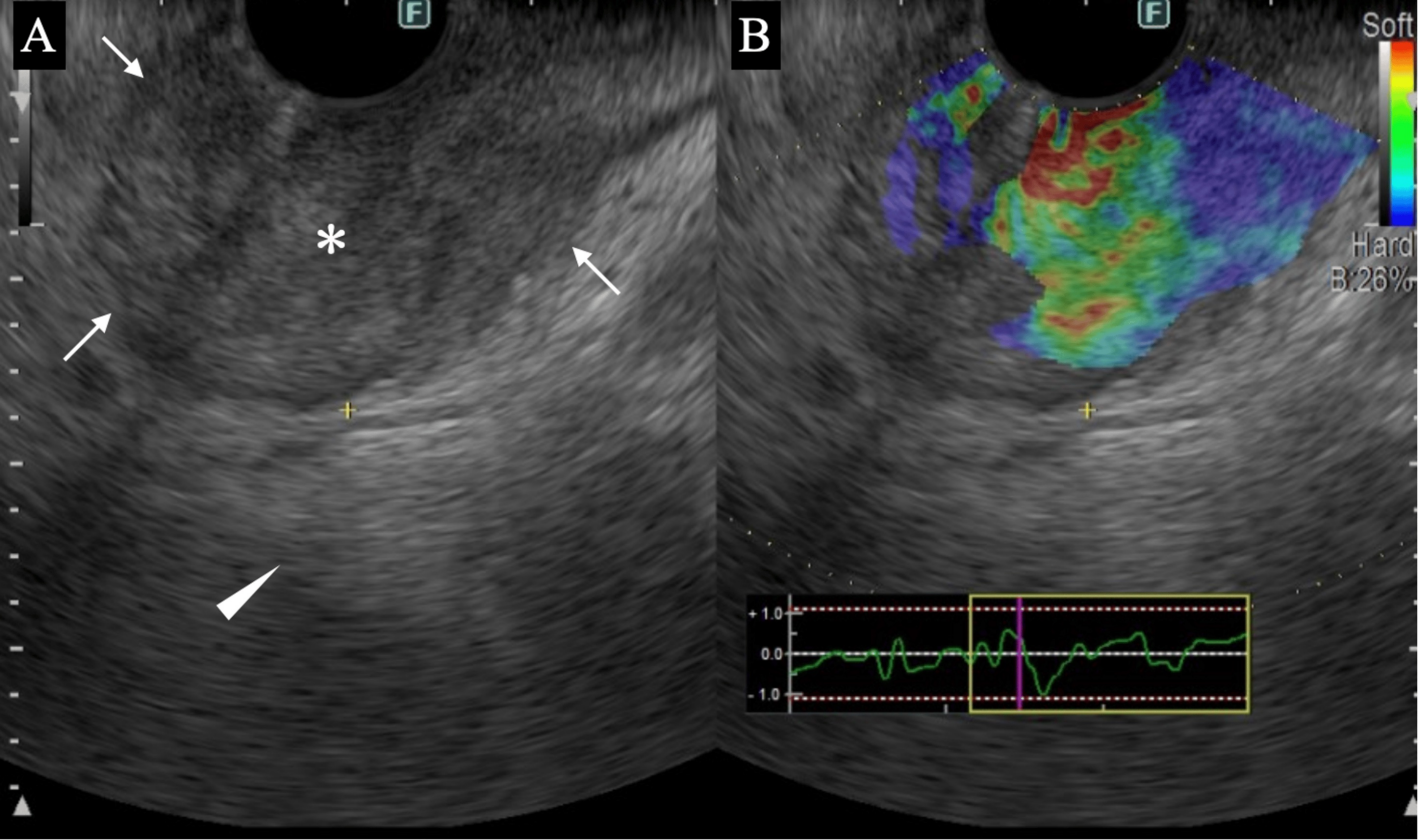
EUS findings (A) EUS showed a circumscribed oval mass (arrows) with internal high echoes (asterisk) and enhanced posterior echoes (arrowhead). (B) Elastography showed no blue parts at least in the mass center. EUS: endoscopic ultrasonography
